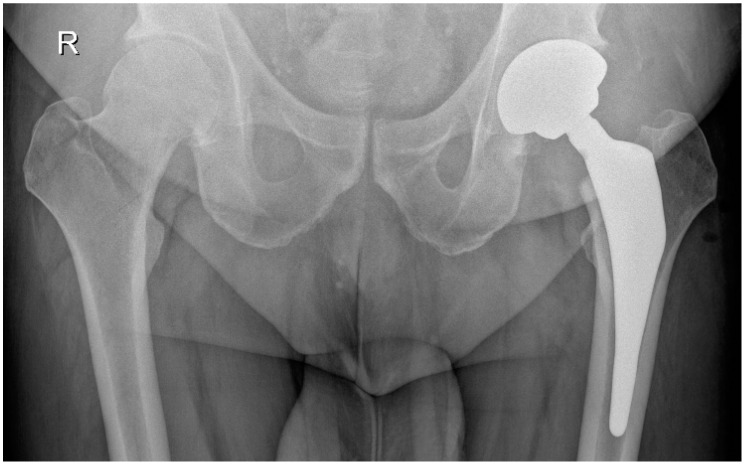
Postoperative X-ray of the same patient after a custom-made THA on the left side.
